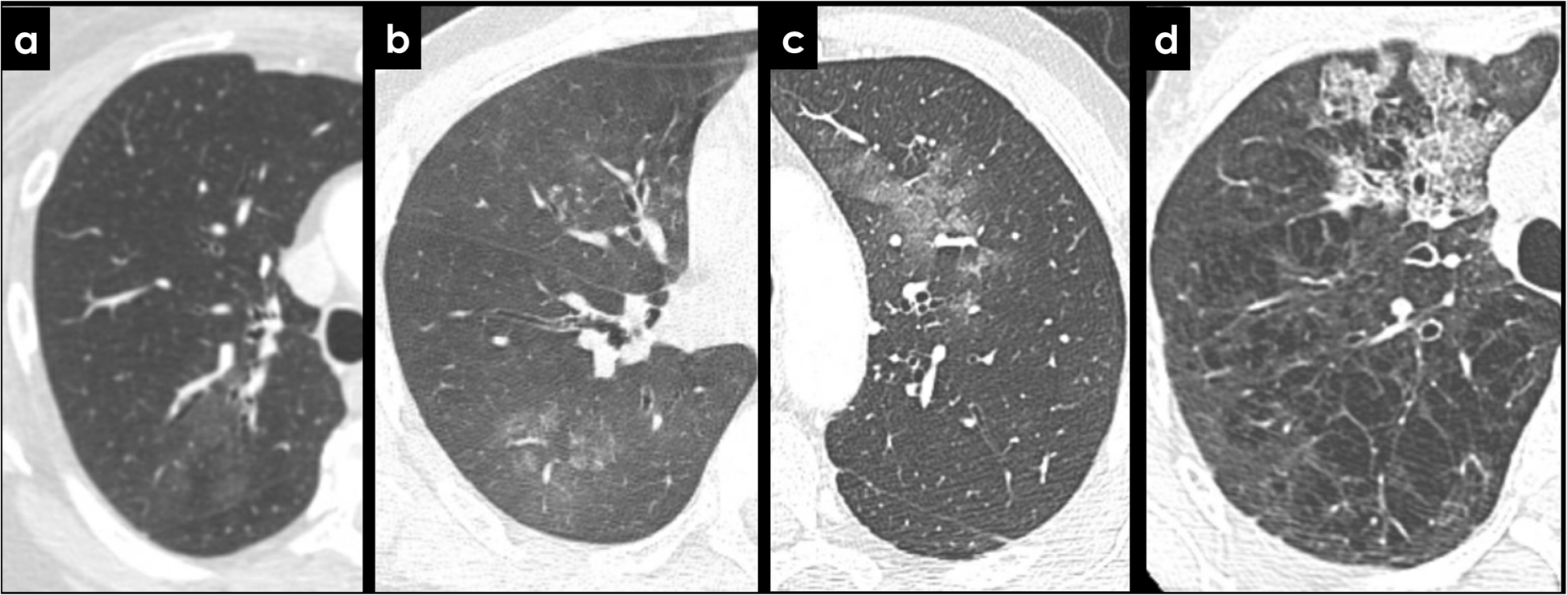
CT findings of pulmonary hemorrhage. Hazy ground-glass infiltrates (a, b, c) representing intra-alveolar hemorrhage in patients with hemoptysis. Also note endobronchial filling in b and crazy paving pattern in d
